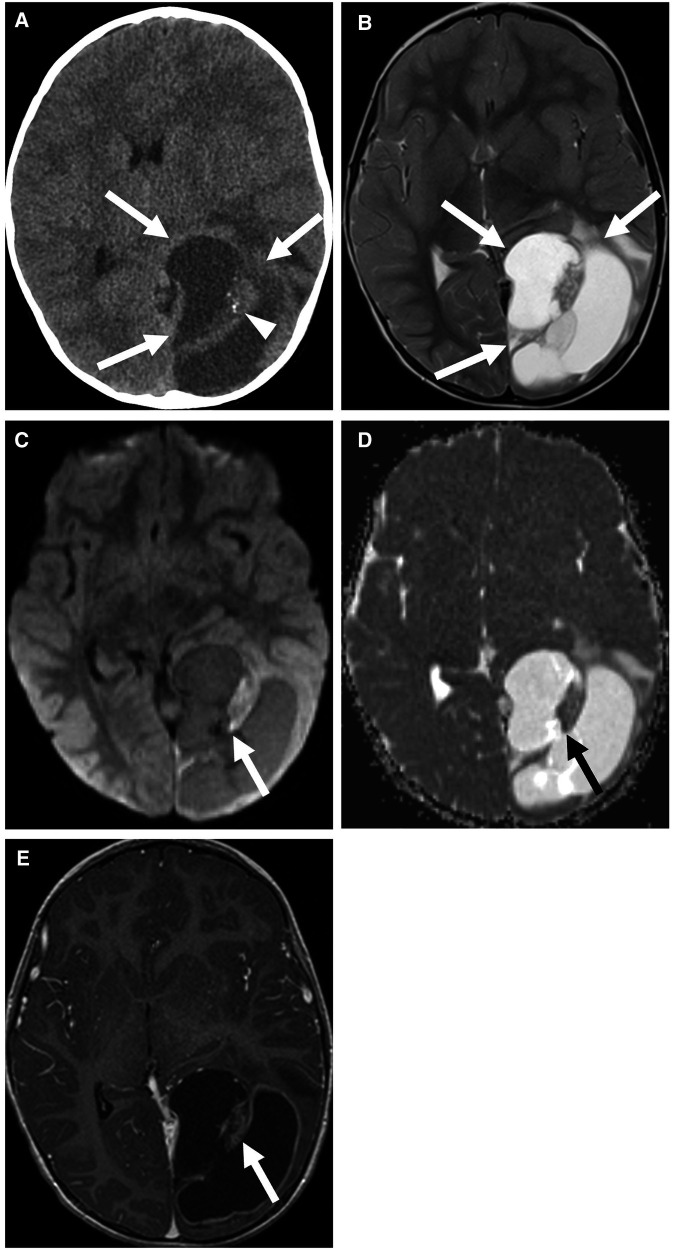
Supratentorial ependymoma, ZFTA fusion-positive in a 3-year-old boy. Non-contrast CT (A) and axial T2 (B) images show a cystic and solid mass centered in the left occipital lobe (arrows) with calcification of the solid component (arrowhead). The solid component of the mass shows mild diffusion restriction (C,D) and enhancement (E) (arrows). There is also an associated mild rightward shift of the midline.
